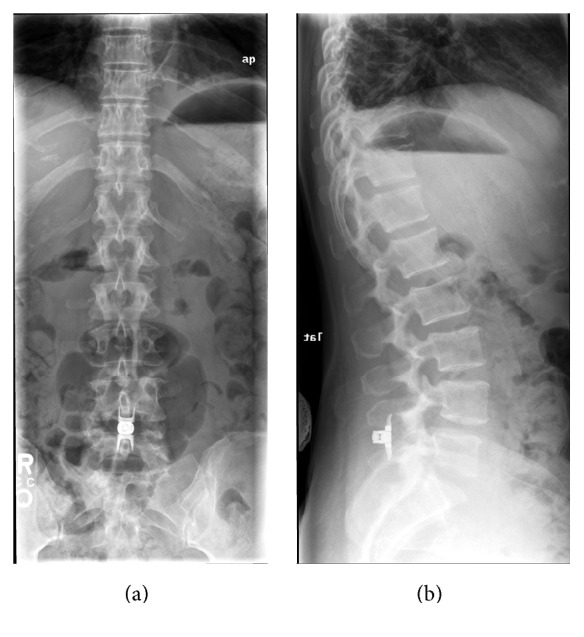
Anteroposterior (a) and lateral (b) plain radiographic images showing proper anatomical positioning of the Superion spacer* in situ*.
